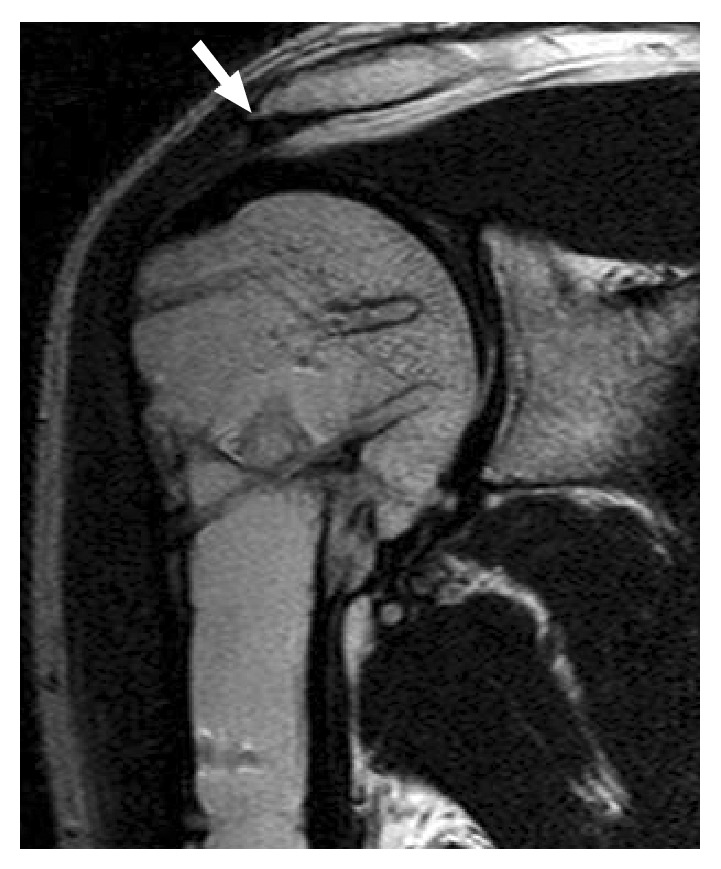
Although no fluid collection is seen in the subacromial bursa, the presence of a newly formed spur formation (arrow) is confirmed at the lateral margin of acromion (oblique coronal T2-weighted MR image).
